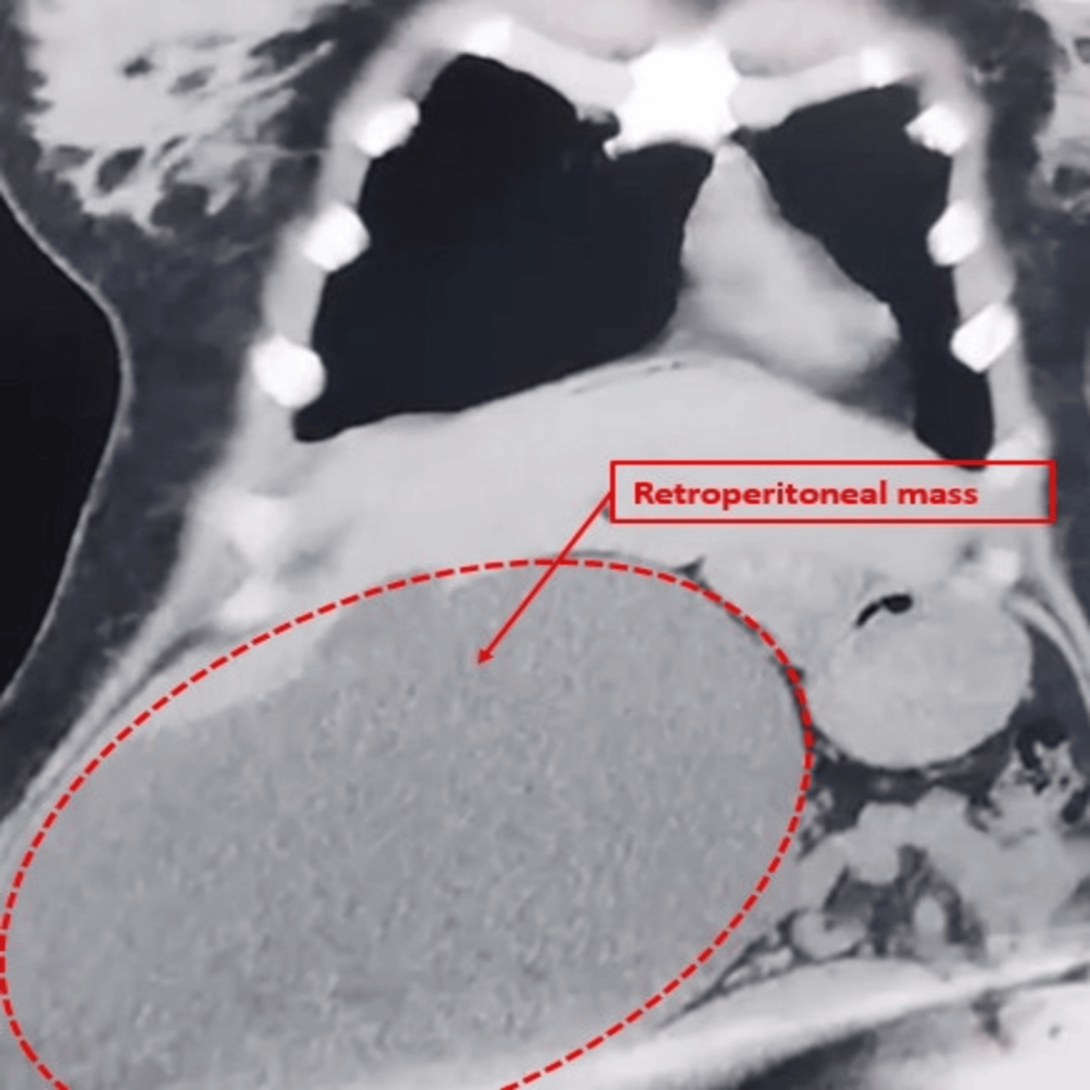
Coronal CT scan showing a giant retroperitoneal cystic mass Coronal non-contrast CT scan of the abdomen revealing a large, well-circumscribed hypodense retroperitoneal cystic mass (outlined with a red dashed line). The lesion occupies the right flank, displacing the right kidney medially and superiorly. No internal septations, solid components, or calcifications are visible. These findings are consistent with a retroperitoneal cystic lesion; differential diagnoses include hydatid cyst, lymphangioma, or adrenal pseudocyst. CT: computed tomography
